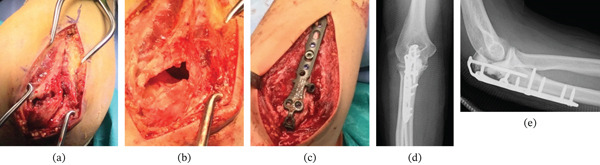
Intraoperative macroscopic findings and postoperative plain radiographs. (a) The tumor was exposed through the fracture site. Adequate visualization was obtained via the displaced fracture segment without additional cortical windowing, facilitating thorough intralesional curettage. (b) The tumor cavity after curettage. The articular cortical bone was clearly visualized through the fracture gap. Accurate anatomical reduction was achieved during temporary fixation prior to bone grafting. (c) Intraoperative photograph after definitive internal fixation using an olecranon plate, demonstrating stable fixation following curettage and reconstruction. (d) Postoperative anteroposterior plain radiograph. (e) Postoperative lateral radiograph showing satisfactory restoration of the articular surface.
